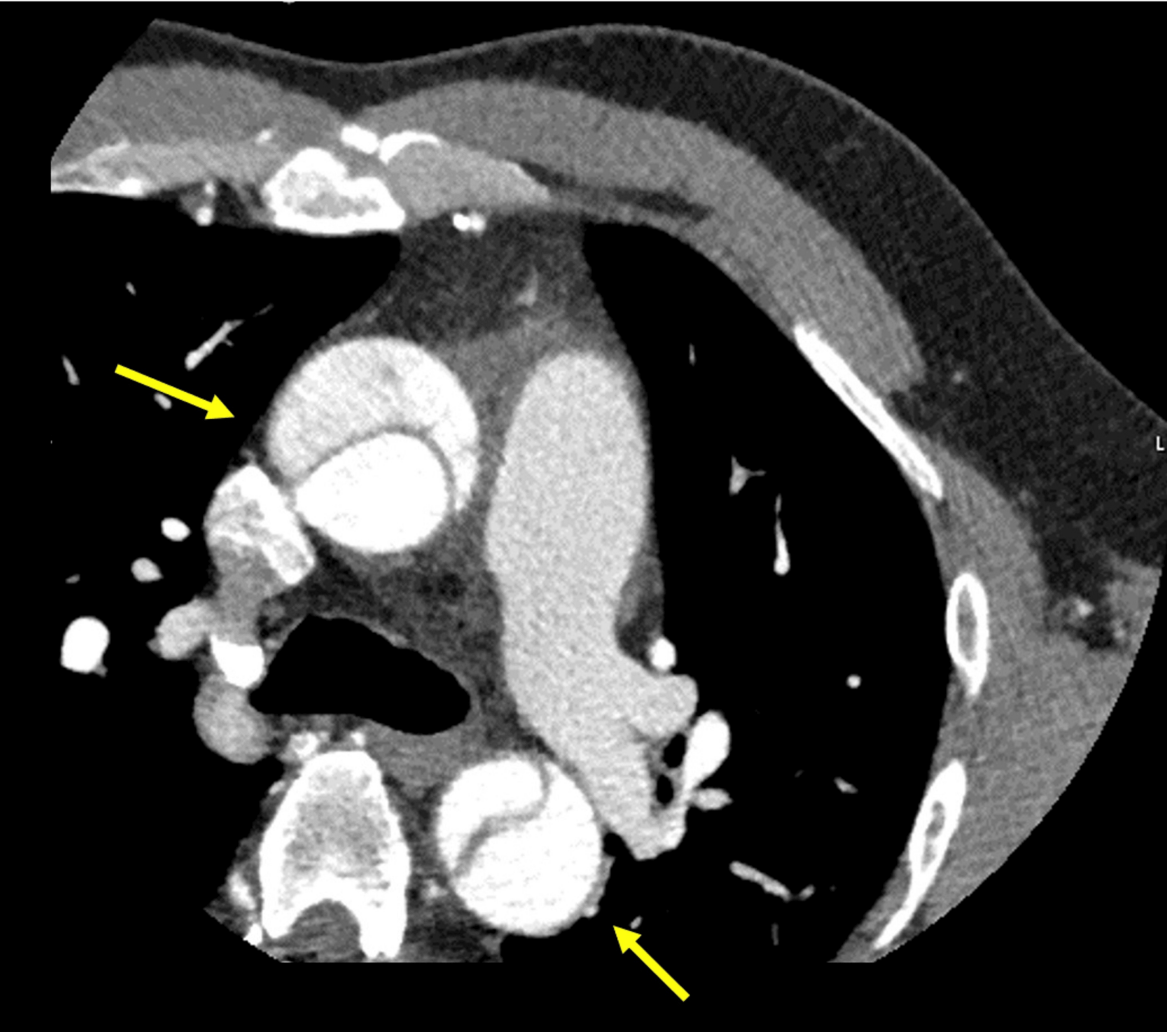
Chest contrast computed tomography (CT) scan results on admission The chest contrast CT shows arterial dissection extending from the ascending aorta to the aortic arch (arrowheads).
